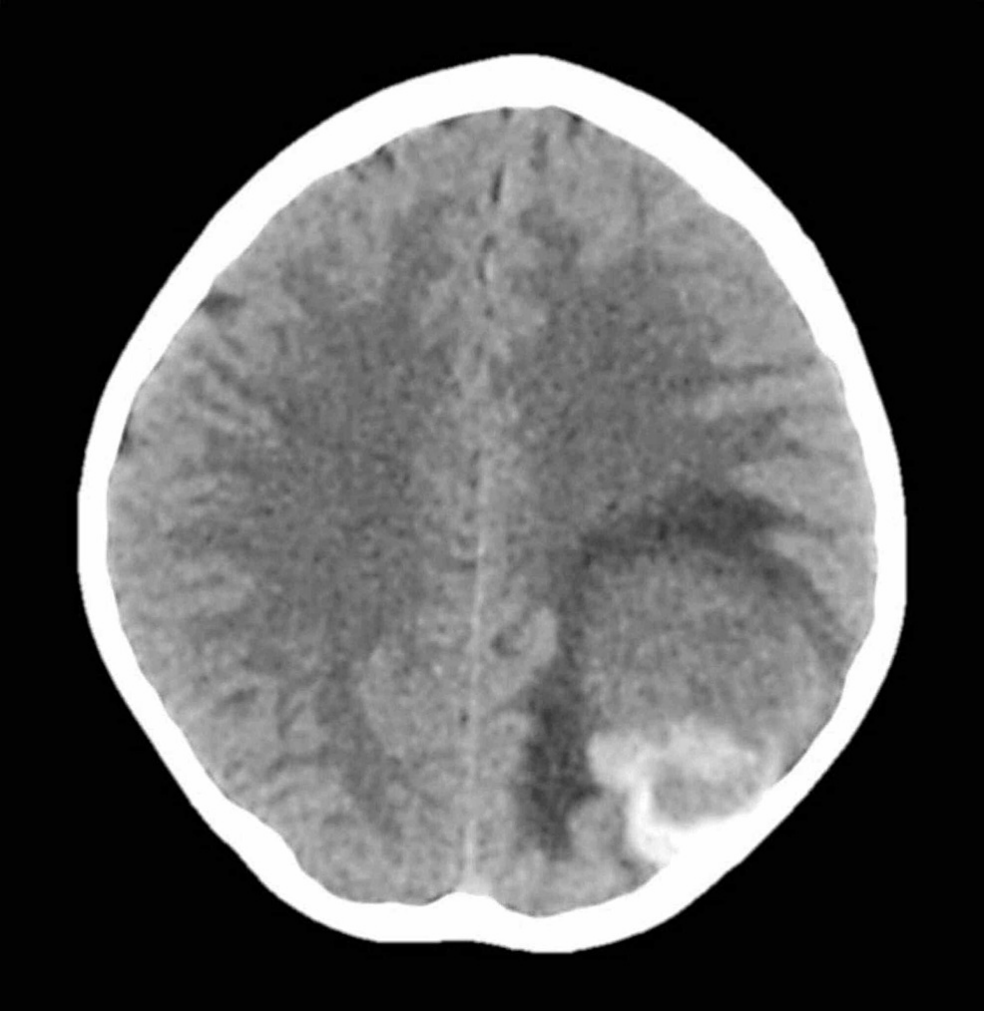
Urgent CT scan of the brain. The urgent CT scan revealed rebleeding in the previous hemorrhage site with signs of resorption of the first intracranial hemorrhage.
